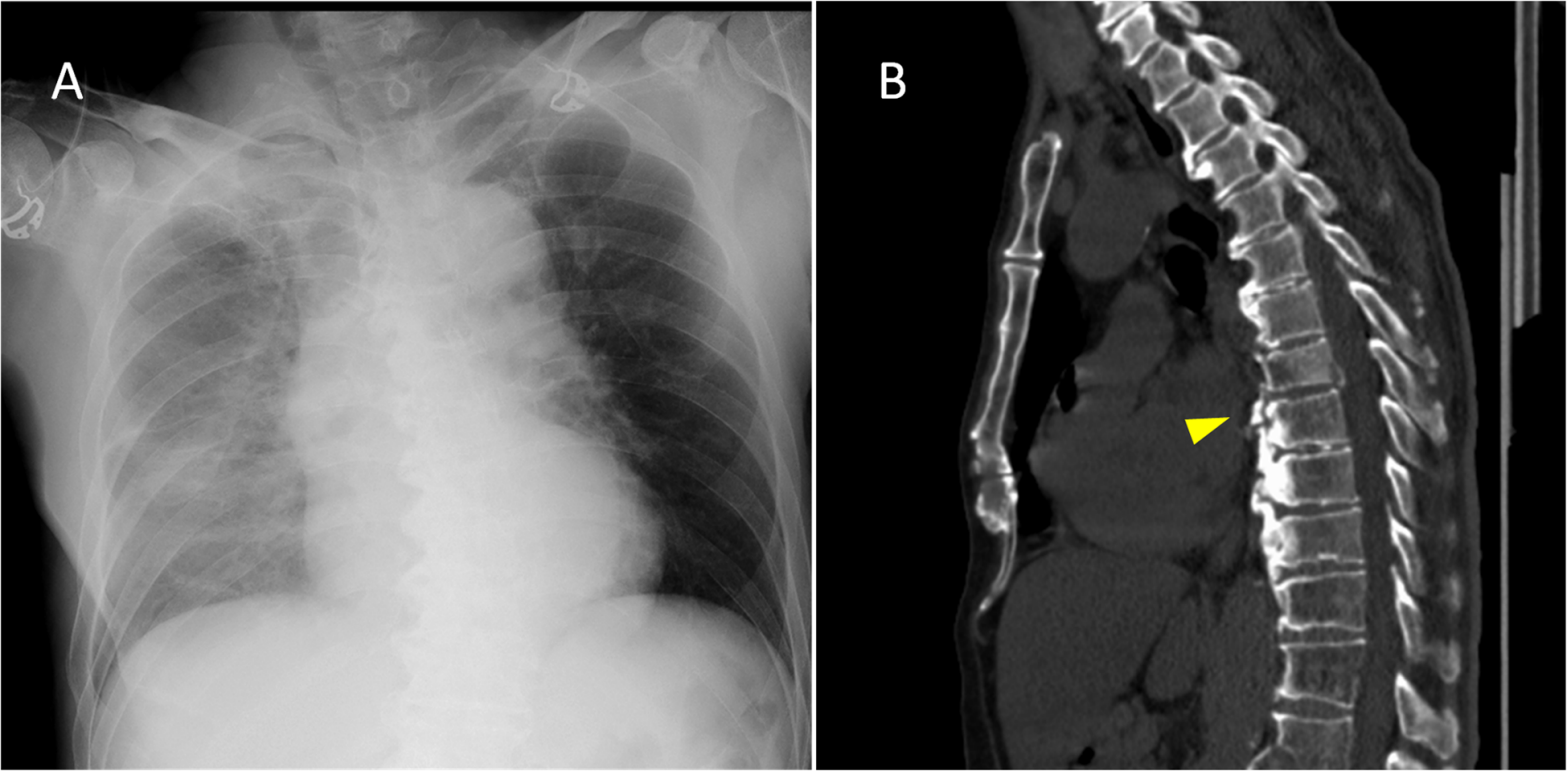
Images from Case 2: a Chest radiography shows a diffuse opacity of the right chest, and b computed tomography shows a T7-burst fracture (arrow head) and L1 fracture
